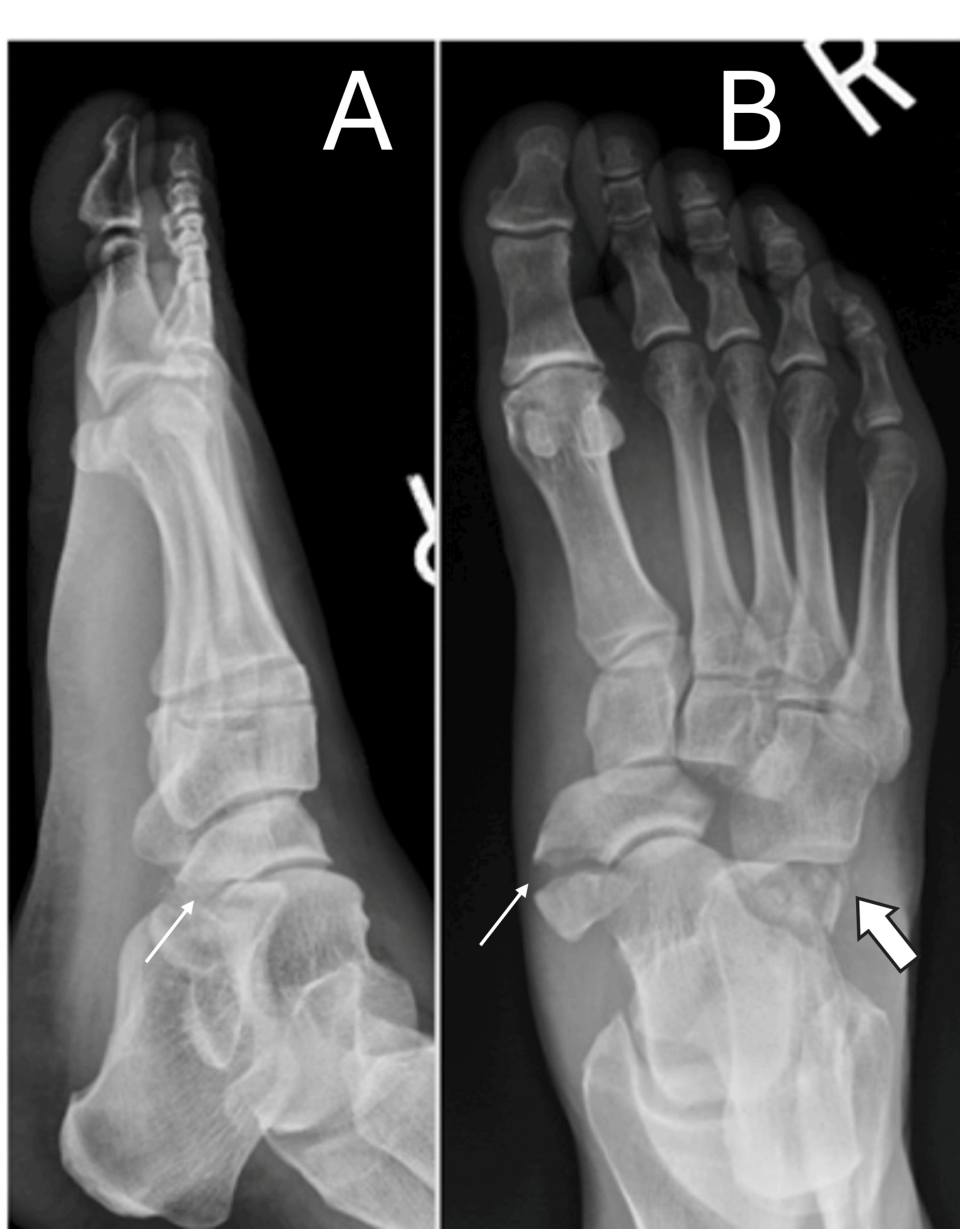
AP (B) and lateral (A) radiographs demonstrating naviculocuneiform dislocation with associated navicular fracture (small arrow (B)). Calcaneocuboid dislocation with a fracture of the anterior process of the calcaneus (small arrow (A), large arrow (B)).
